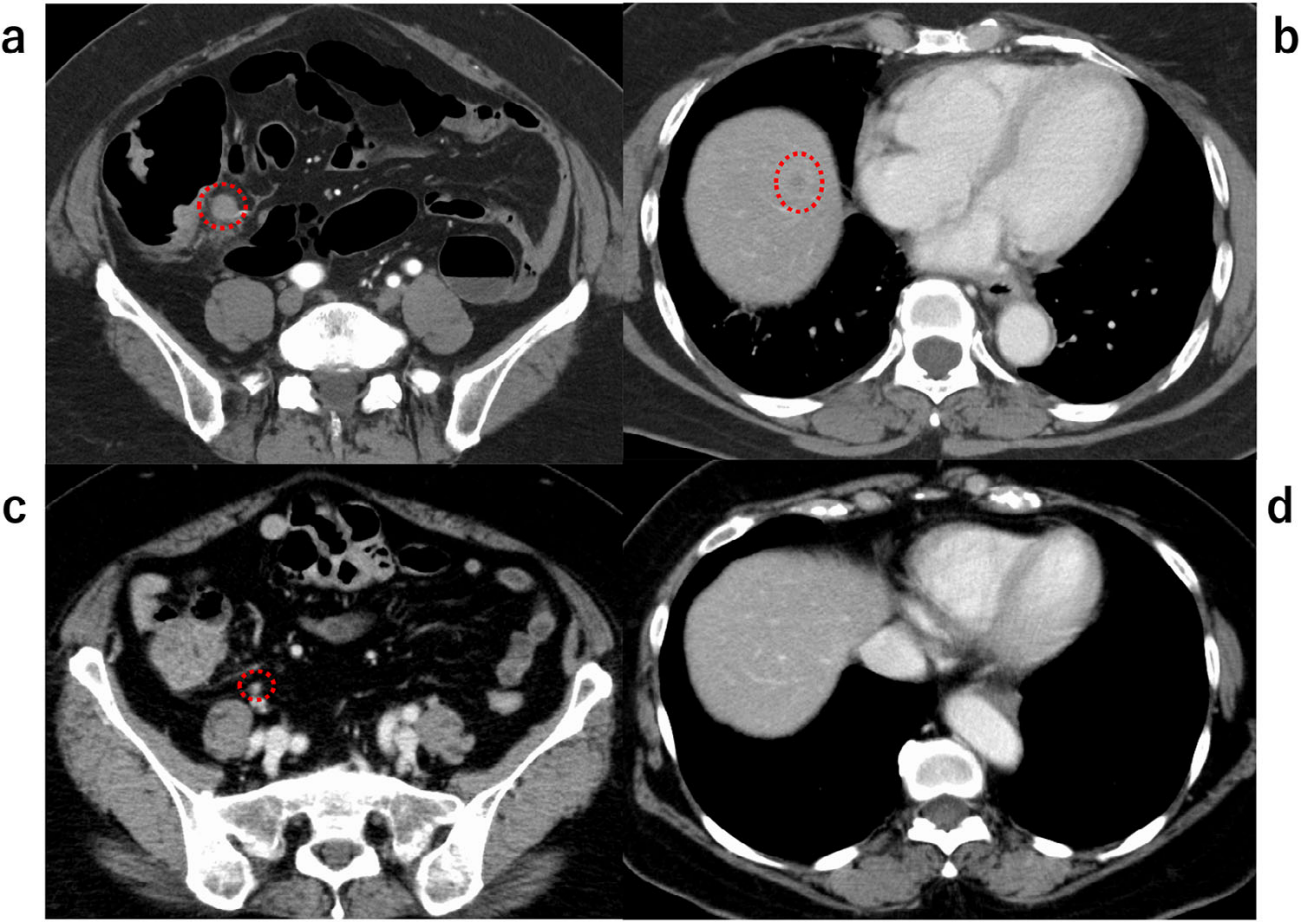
Comparison of lymph node and liver metastasis on computed tomography (CT) scan before and after chemotherapy. (a) Lymph node metastasis before chemotherapy. (b) Liver metastasis before chemotherapy. (c) Lymph node metastasis after chemotherapy, showing regression. (d) Liver metastasis after chemotherapy, showing regression.
